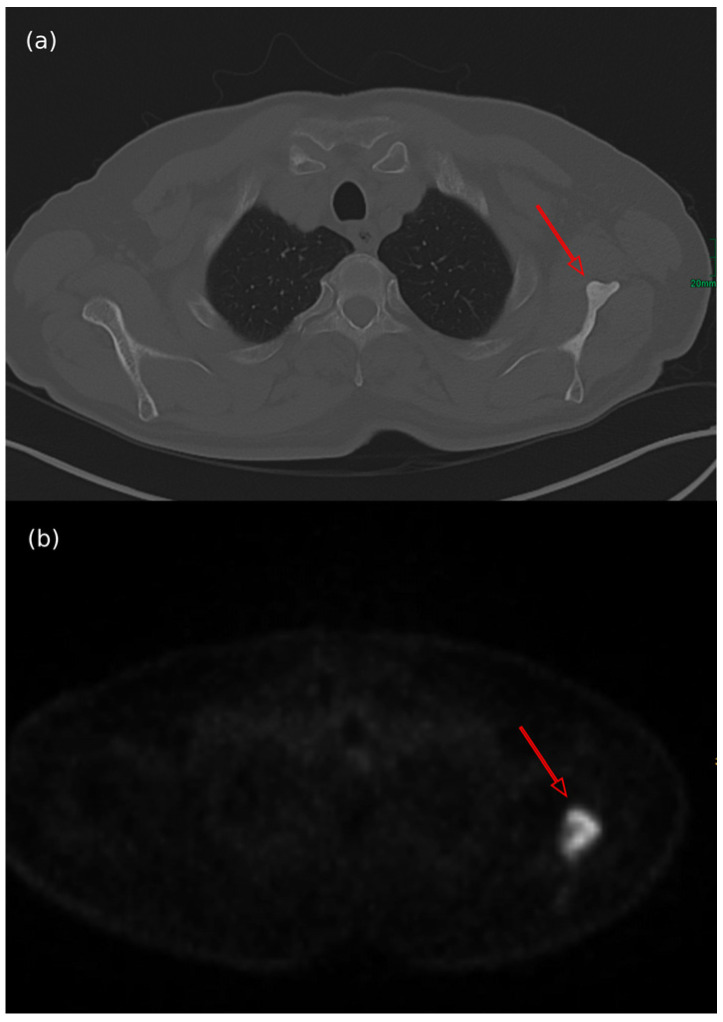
Osteoblastic bone metastasis from Merkel cell carcinoma. (a) Axial CT image showing an osteoblastic lesion in the left scapula (red arrow). (b) Corresponding PET scan reveals FDG uptake in the same region (red arrow), consistent with metabolically active metastatic disease.
